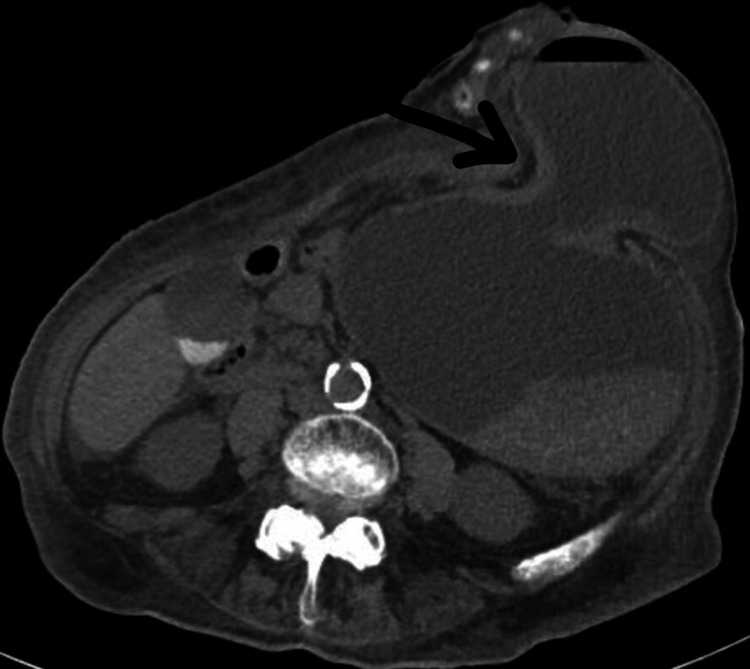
CT abdomen and pelvis on day one. Arrow showing parastomal hernia containing a portion of the distal stomach. CT: computerized tomography.
